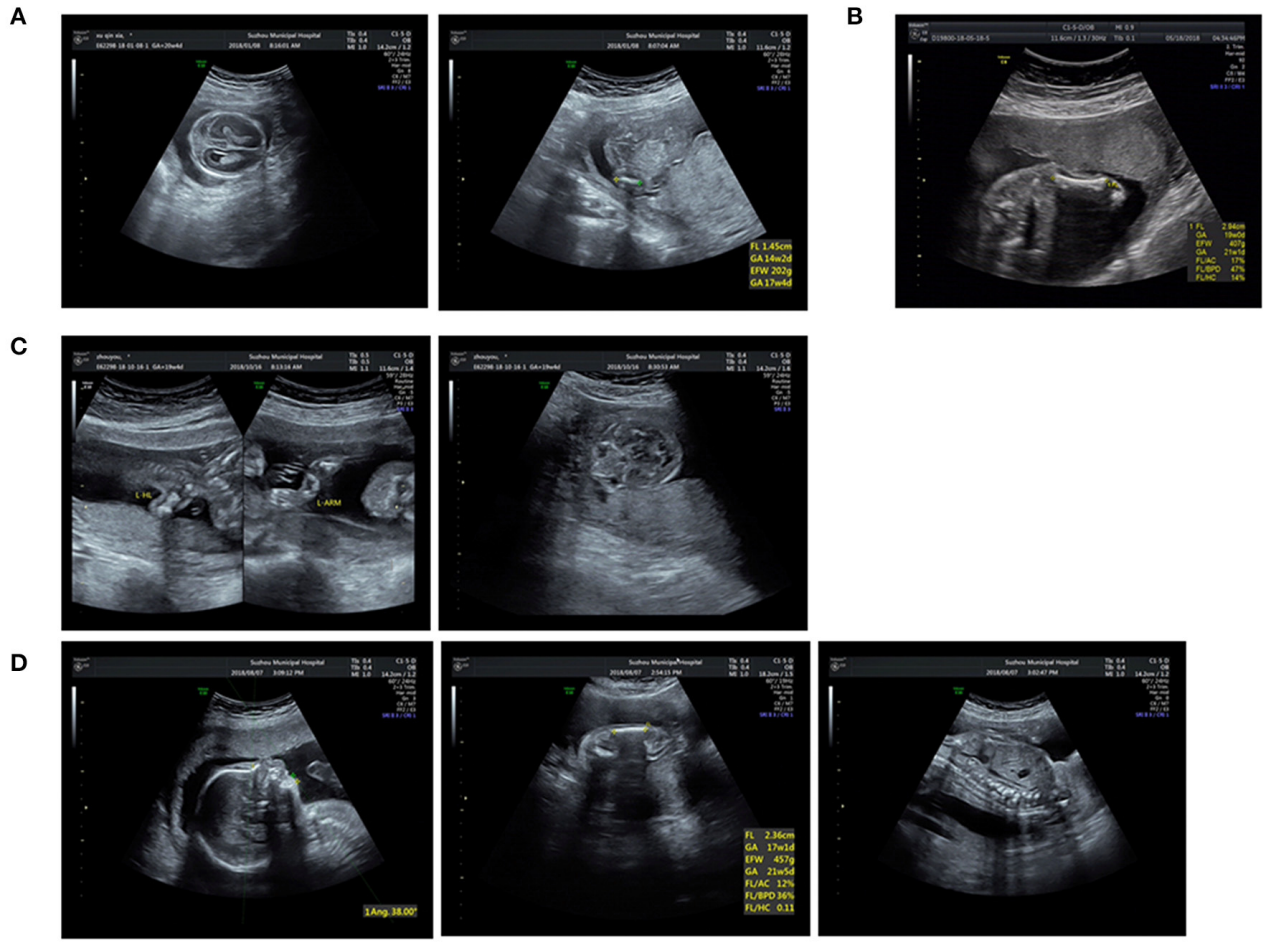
Ultrasound pictures of cases 4, 6, 8, and 10: (A) Short femur and cloverleaf skull of P4. (B) Short femur of P6. (C) Short long bones and wide orbilal septum of P8. (D) Short limbs, micrognathia, and spine misaligned of P10.
